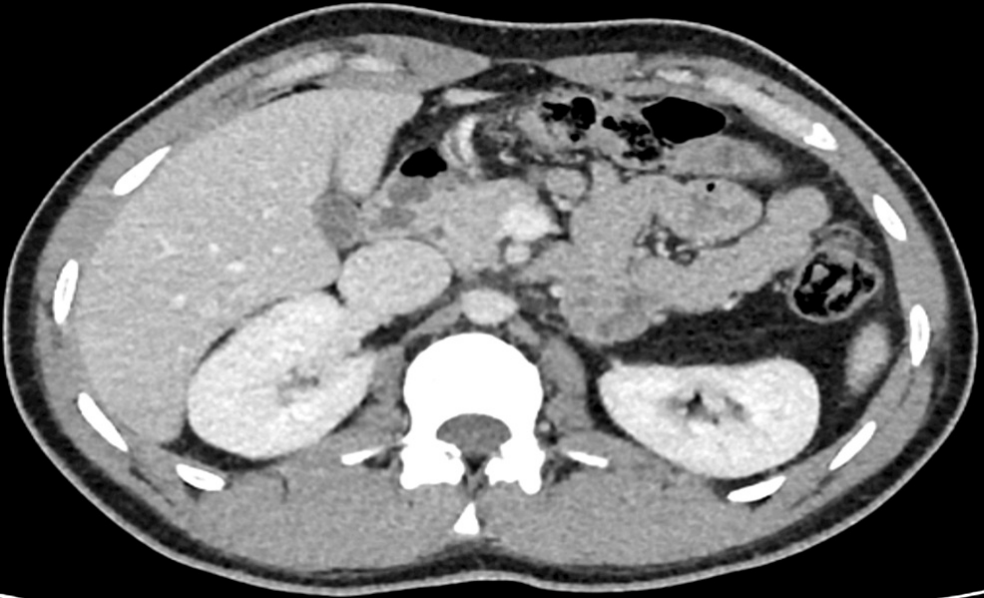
CT after surgical resection of the tumor. Kidneys are of normal enhancement with no stone, mass or hydronephrosis. Right adrenal is normal, while the left adrenal is resected with no obvious residual masses in the surgical bed.
